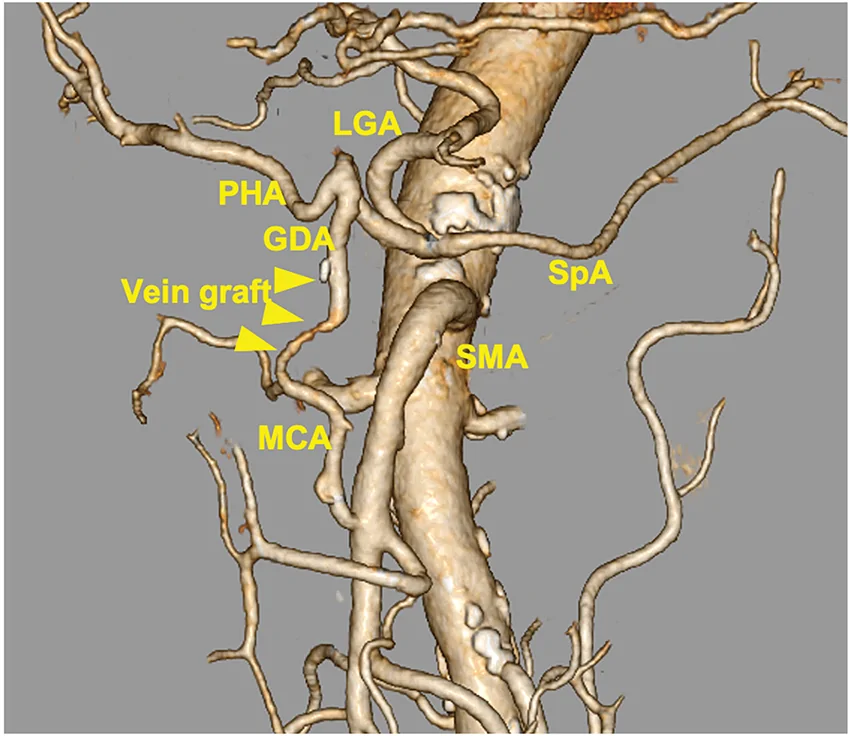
3D CT angiography on POD 7. The vein graft is indicated by yellow arrowheads. Although focal poor enhancement is observed in a portion of the graft, hepatic arterial flow is preserved. GDA, gastroduodenal artery; LGA, left gastric artery; MCA, middle colic artery; PHA, proper hepatic artery; SMA, superior mesenteric artery; SpA, splenic artery
